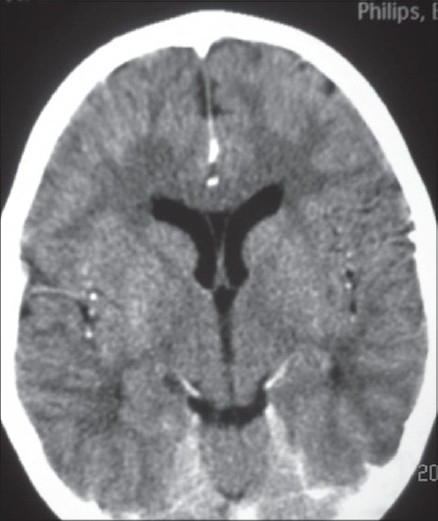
CT brain showing ill-defi ned hypodensities in subcortical white matter in both parietal lobes.
